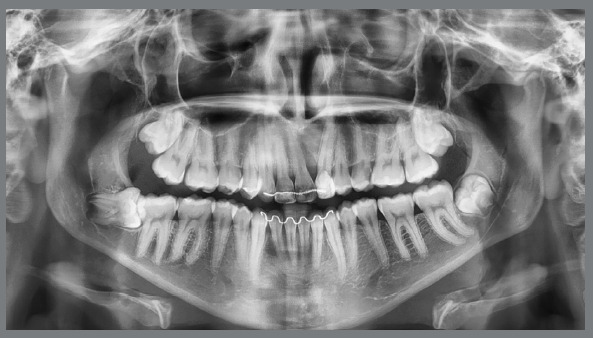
26-month retention panoramic radiograph.
